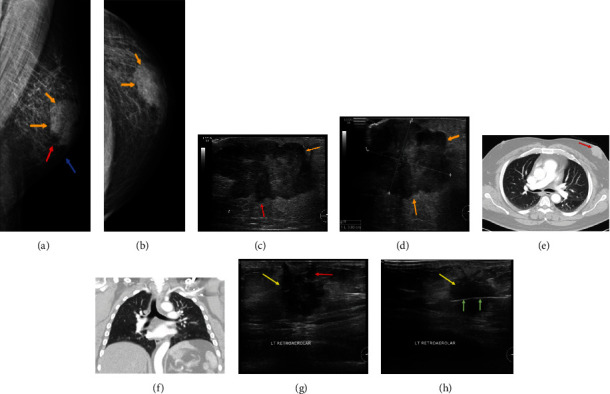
Left mammogram, ultrasound of the left breast, and chest CT of a male patient with malignant breast cancer. (a) MLO view and (b) CC view, showing a large dense mass (yellow arrow) in the retroareolar and upper location. The mass has irregular margins with adjacent coarse trabeculae (red arrow) and mild skin thickening (blue arrow). No microcalcification or skin retraction was observed. (c, d) Complementary ultrasound of the left breast revealed a huge mass (yellow arrow) with lobulated contours and heterogenous echoes. The lesion measured ~3.8 × 3.0 cm, was located at 1-2 o'clock position, and appeared to invade the adjacent parenchyma (red arrow). (e) CT images (lung window) of the first case showing a mass of the left breast (red arrow). (f) There was no evidence of metastasis to the lungs or mediastinum. (g, h) Show the ultrasound-guided left breast biopsy of the second malignant case. (g) A huge hypoechoic mass (yellow arrow) with lobulated contours. This is located at 1 to 2 o'clock position and appears to be invading the adjacent parenchyma (red arrow). (h) Shows the tumor mass (yellow arrow) and biopsy needle (green arrow).
